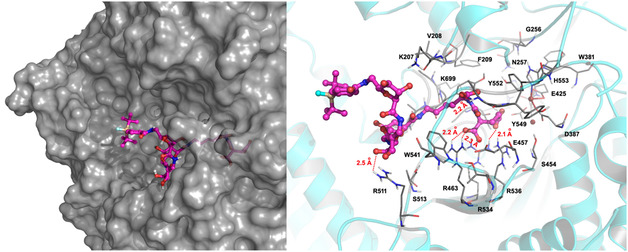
Molecular docking results for 21 docked in PSMA (PDB: 5O5T).
